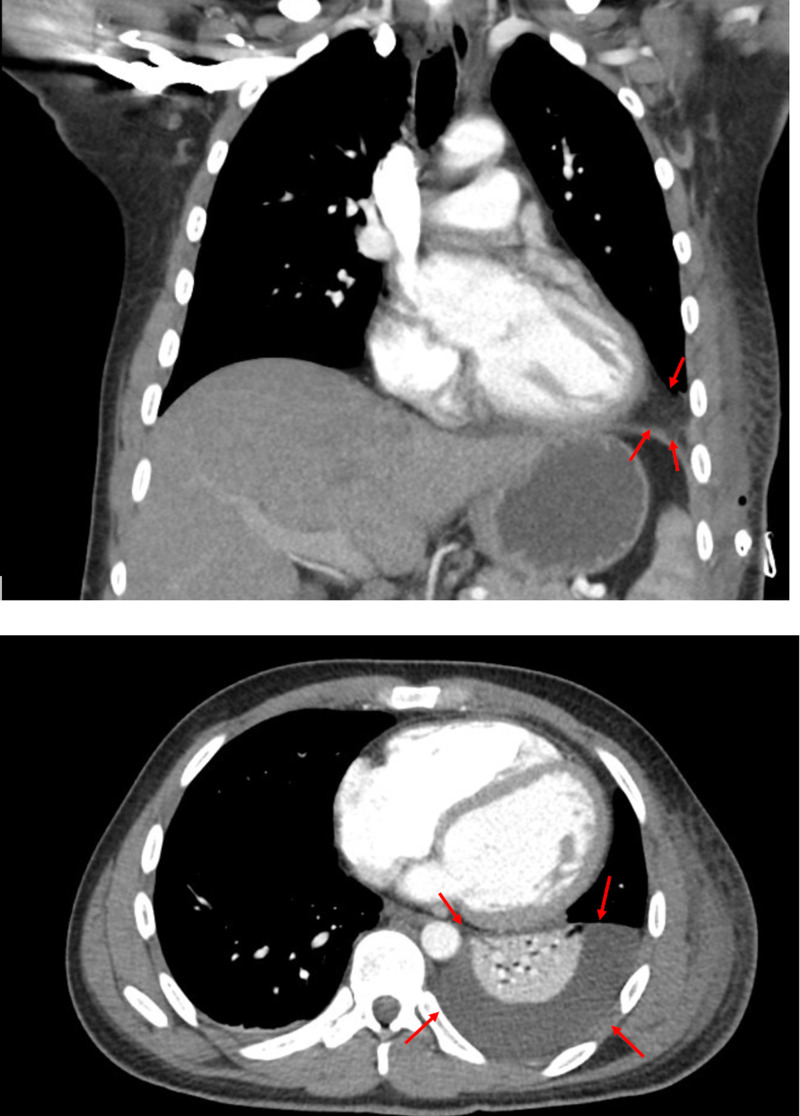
Repeat CT with contrast on day 3 of hospitalization. The initial insult evolved to a small consolidation and pleural effusion which are marked with red arrows in the coronal and axial views on CT scan.
